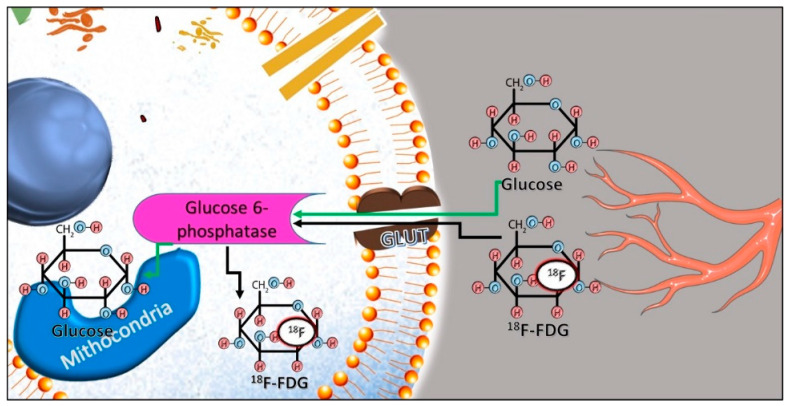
Internalization and phosphorylation of ^18^F-FDG from the bloodstream in cells.
